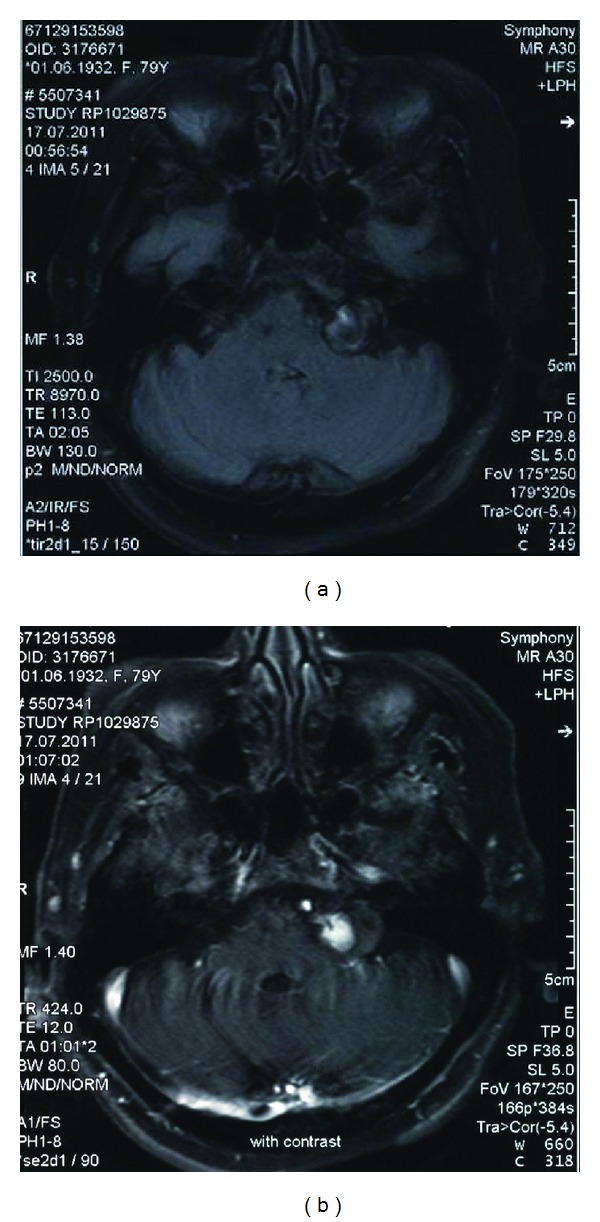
(a) and (b) Axial flair and axial postcontrat T1 sequences of cranial magnetic resonance imaging show a lesion with heterogeneous intensity with contrast enhancement.
